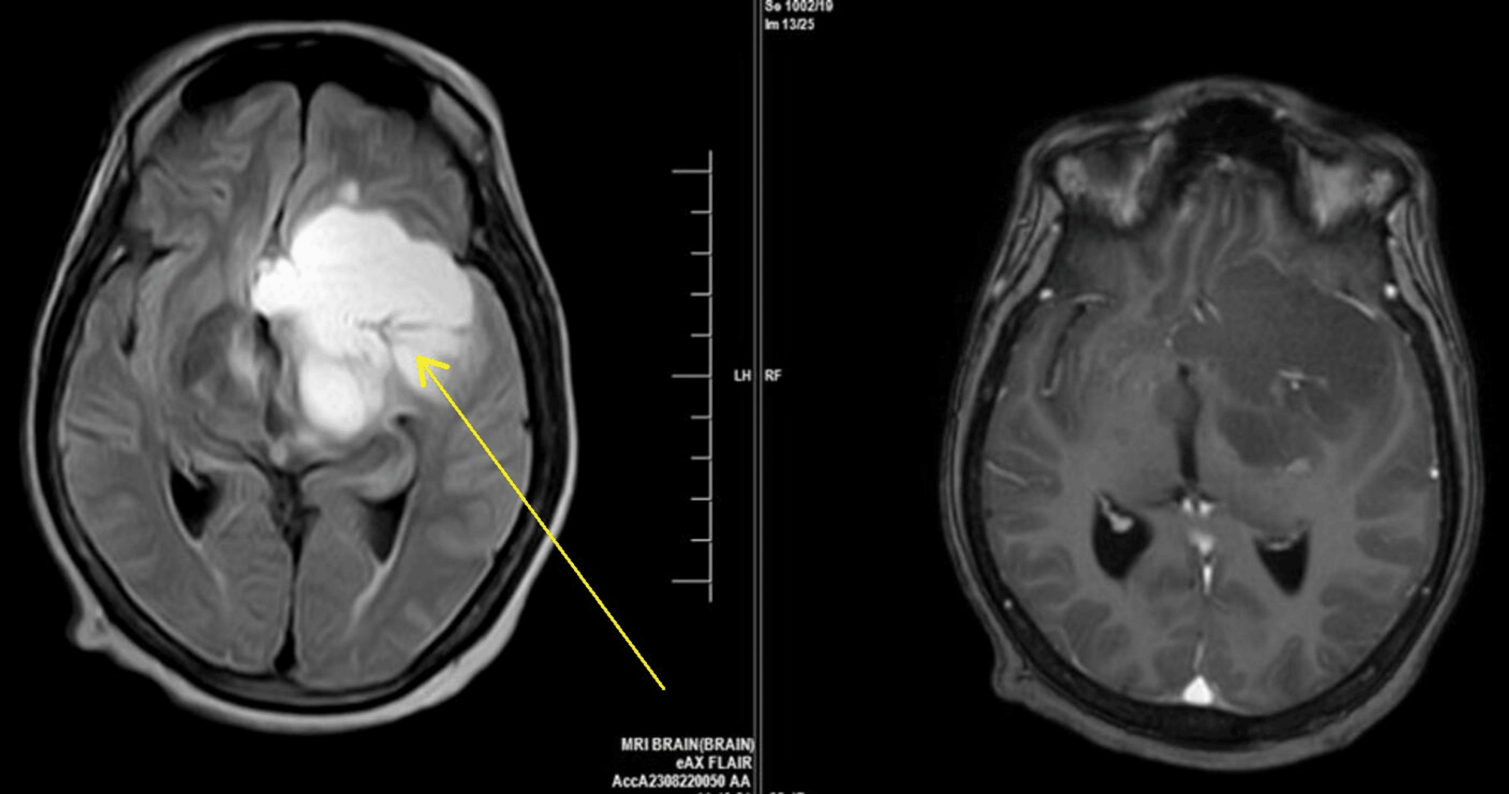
MRI Brain suggestive of lesion compressing the interpeduncular and left temporal regions (Yellow arrow).
